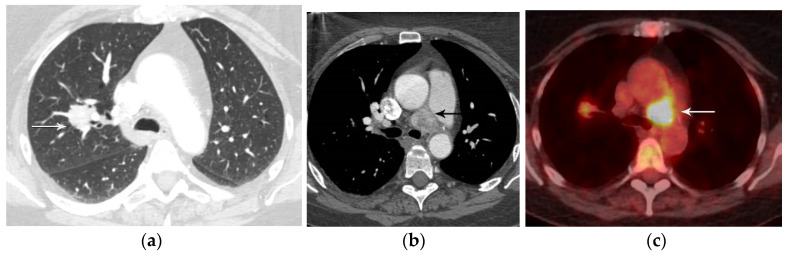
Paraganglioma mimicking N3 nodal metastasis. (a) CT shows a right upper lobe primary lung cancer (arrow). (b) Contrast-enhanced CT shows enhancing soft tissue in the left lower paratracheal region (arrow). (c) PET/CT shows the left mediastinal soft tissue is FDG avid, suspicious for N3 contralateral mediastinal nodal metastasis. Biopsy of the left paratracheal mass showed paraganglioma.
